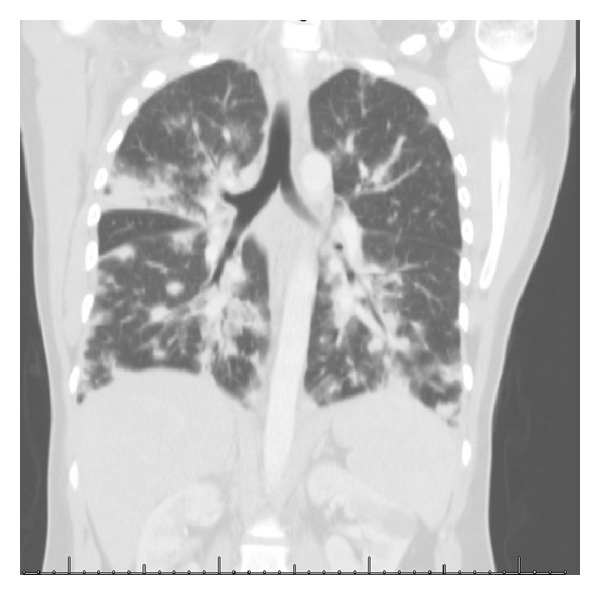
Initial chest CT scan at the ICU, revealing bilateral patchy infiltrates suggestive of lung KS or alveolar hemorrhage.
